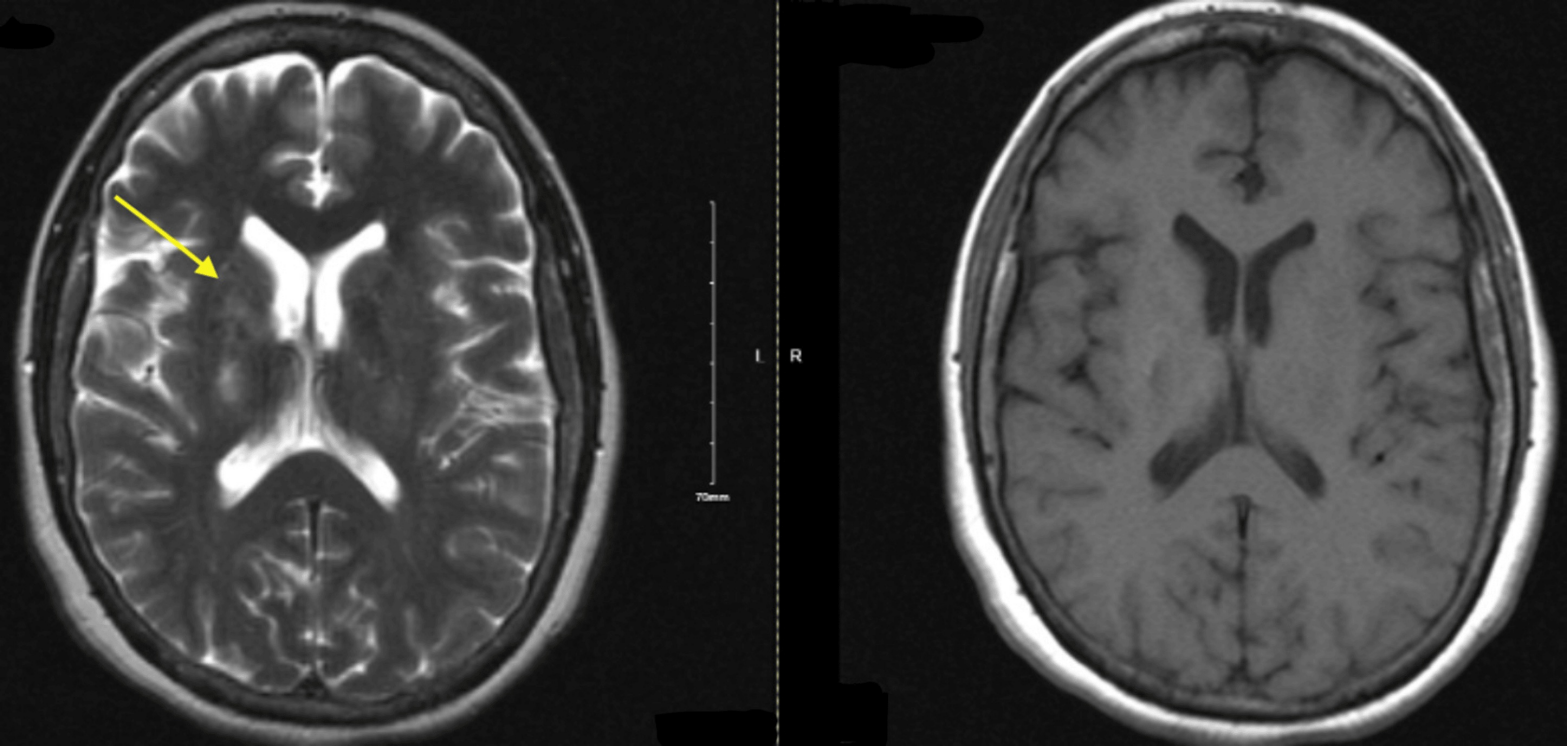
Repeat MRI and T1 with gadolinium. Repeat MRI, performed three months after the second Rituximab dose, showed improvement in lesions involving the right periventricular region, basal ganglia, bilateral thalami, and the posterior limb of the right internal capsule (left image, yellow arrow). Right image is T1 with gadolinium.
